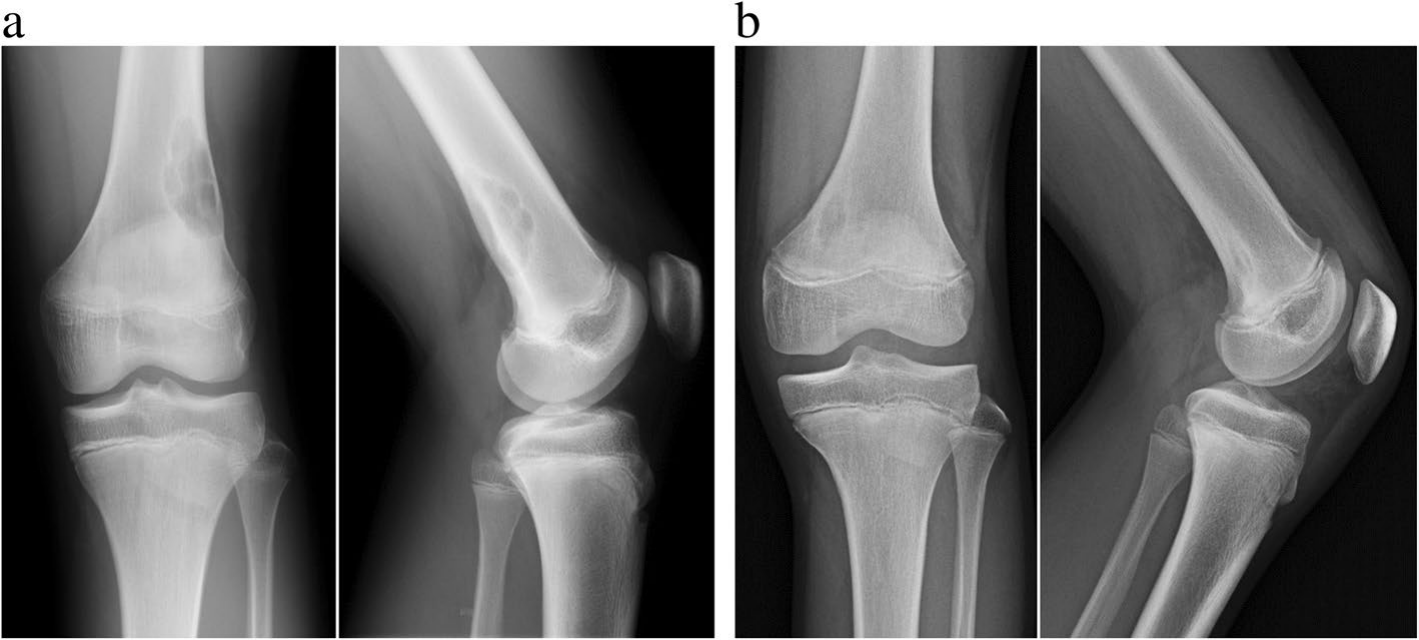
a Representative radiograph of a non-ossifying fibroma. 14-year-old boy with non-ossifying fibroma involving the distal posteolateral portion of the femur. Anteroposterior (left) and lateral (right) radiograph show a well-circumscribed radiolucent lesion involving cortex and medullary bone with lobulated thin sclerotic rim. b Representative radiograph of a fibrous cortical defect. 14-year-old boy with cortical fibrous defect involving the posteomedial cortex of the femur. Anteroposterior (left) and lateral (right) radiograph show an oval, sharply marginated, radiolucent zone in the cortex surrounded by a rim of sclerotic bone
